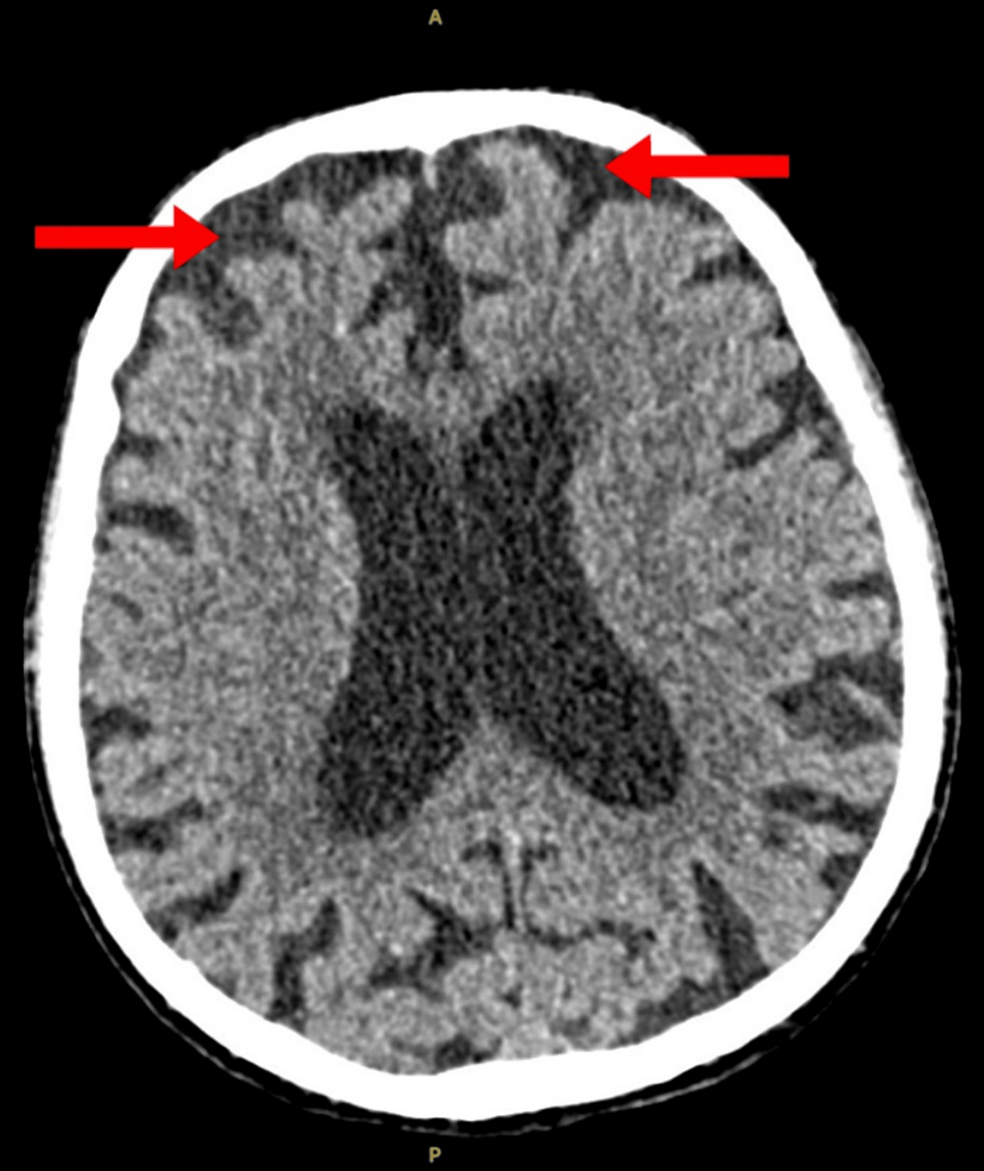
CT brain showing generalized involutional change in proportion to the patient's age (arrows).
